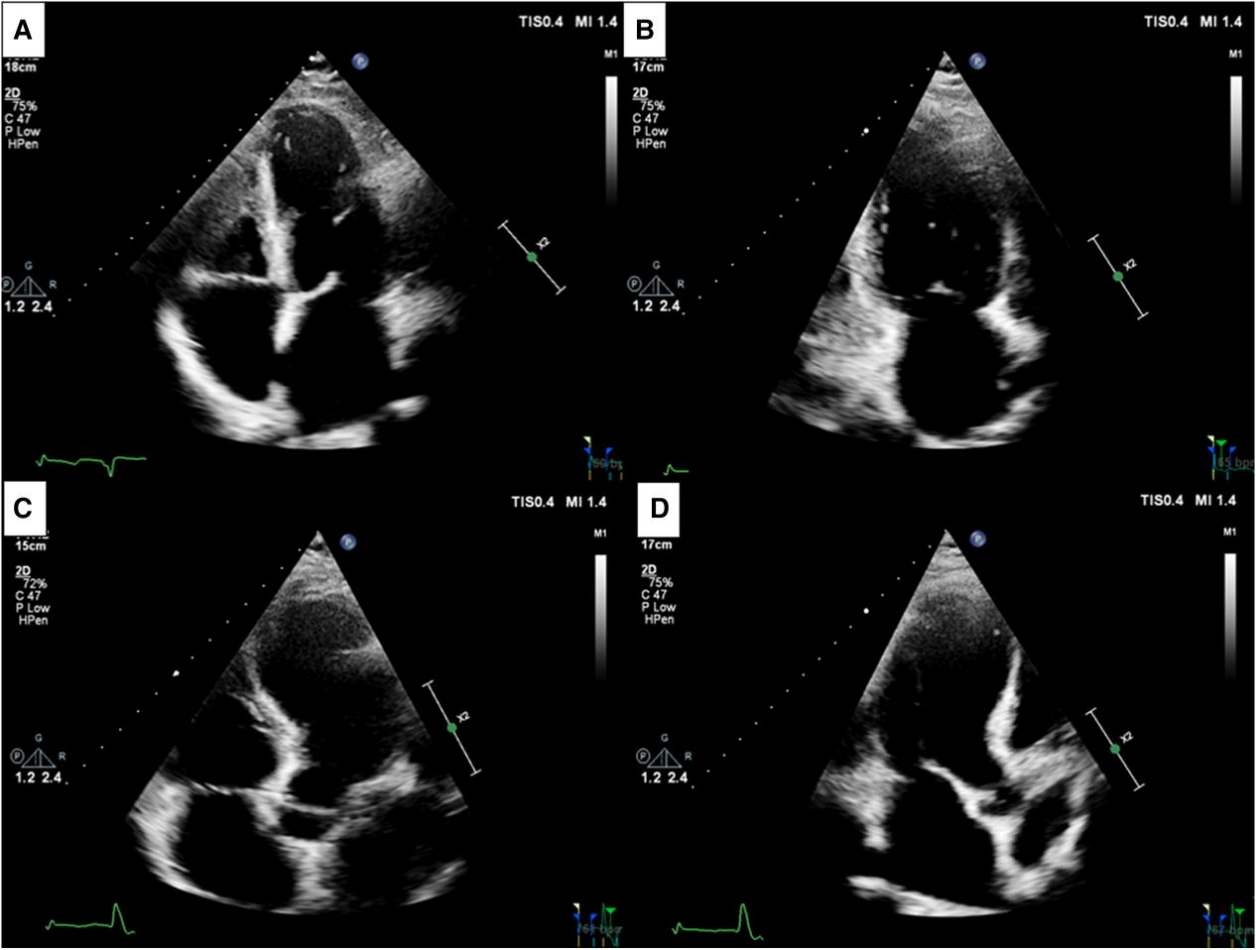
Transthoracic echocardiographic images in the four-chamber (*A*), two-chamber (*B*), five-chamber (*C*), and three-chamber apical views (*D*).
